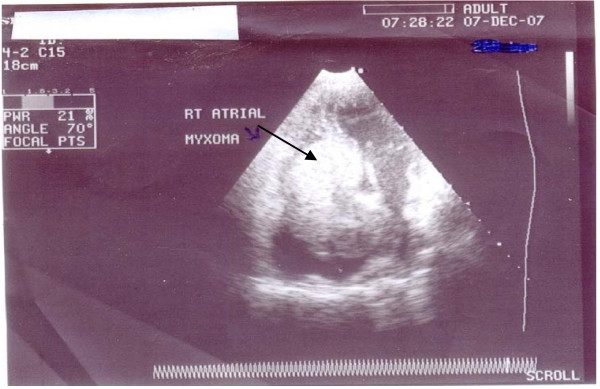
Four-Chamber View of Patient's Transthoracic Echocardiographic Study. This figure shows a large echo dense mass arising from the inter-atrial septum within the right atrium, and prolapsing through the tricuspid valve into the right ventricle. The mass measured 5.3 cm by 4.2 cm.
